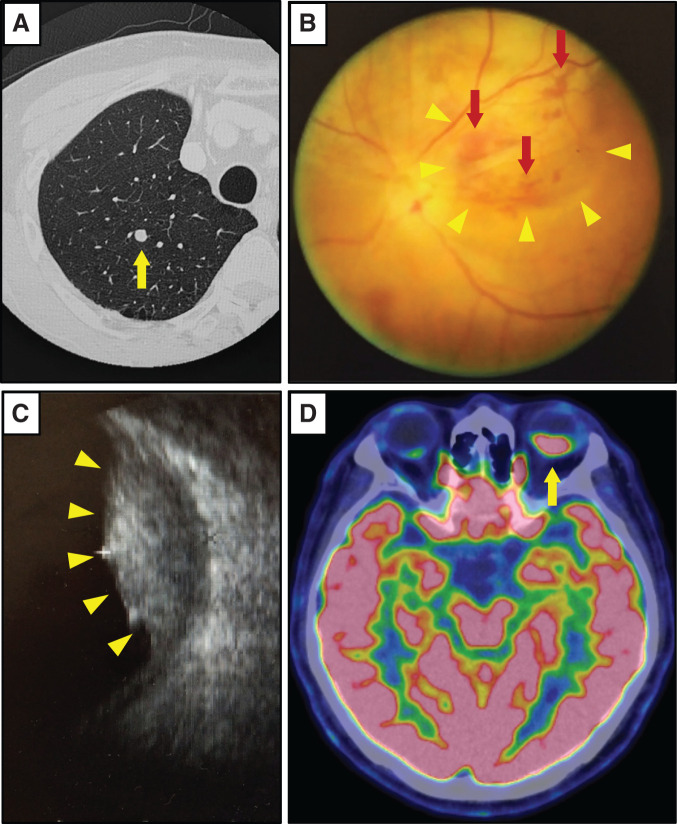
Images when diagnosing choroidal metastasis in the left eye. A 2 mm nodule was detected in the upper lobe of the right lung (A, yellow arrow). Fundus examination revealed a greyish-white tumor shadow (yellow arrowheads) with partial surface bleeding (red arrows) on the outer side of the left optic nerve head (B), and intraocular ultrasound examination detected an 11 mm thick tumor in the choroidal layer (C, yellow arrowheads). PET-CT examination revealed abnormal accumulation in the left choroid (D, yellow arrow).
